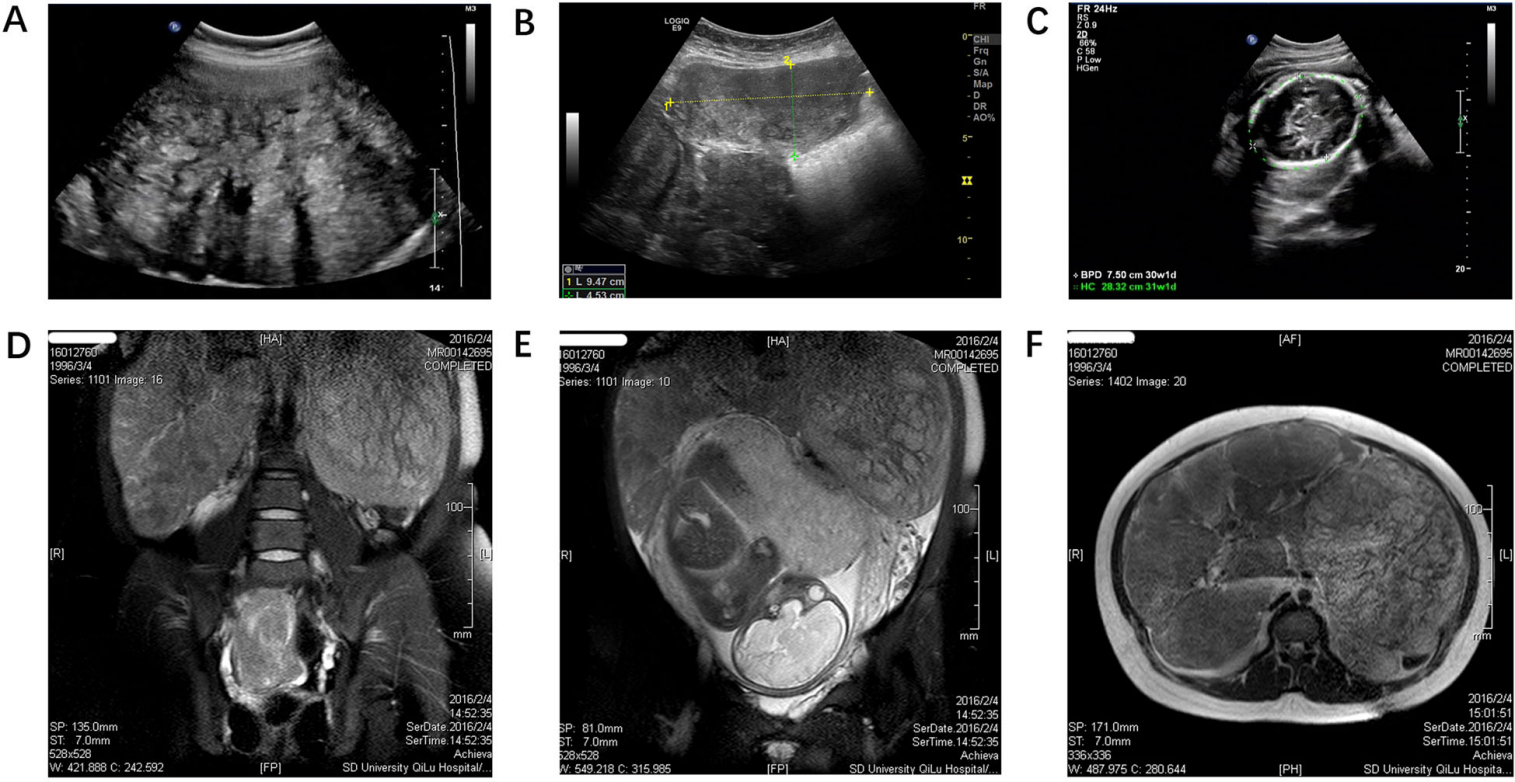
Ultrasound and MRI showed the presence of multiple masses in the pelvis. a, b Ultrasound showed huge mass in the pelvis. c Fetal head in ultrasound. d Multiple huge masses in the pelvis were shown in MRI. e, f The fetal was squeezed by huge masses in MRI
